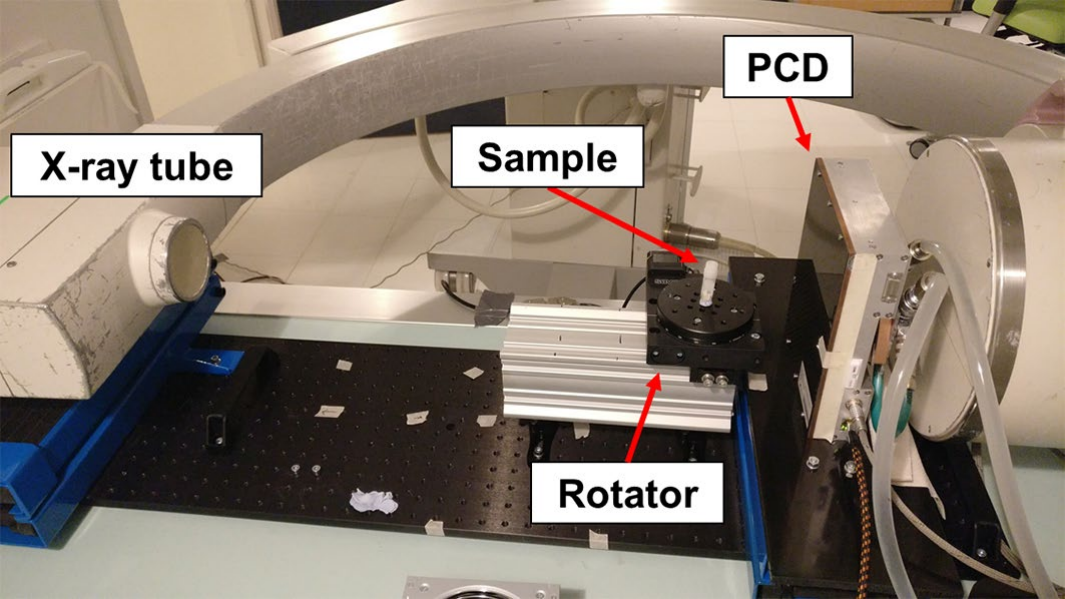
Image of the experimental photon-counting CT setup used in the current study. Photon-counting detector (PCD) is in front of the C-arm’s own detector that is not used in this setup.
